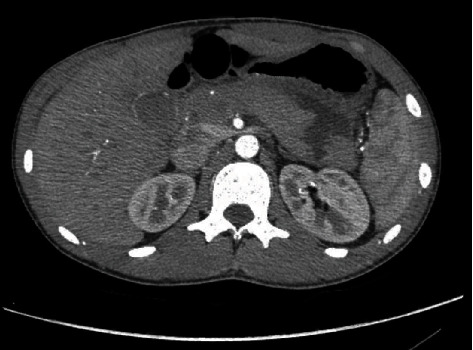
CT scan on the 6th day posttrauma: laceration of the pancreas between the body and tail.
